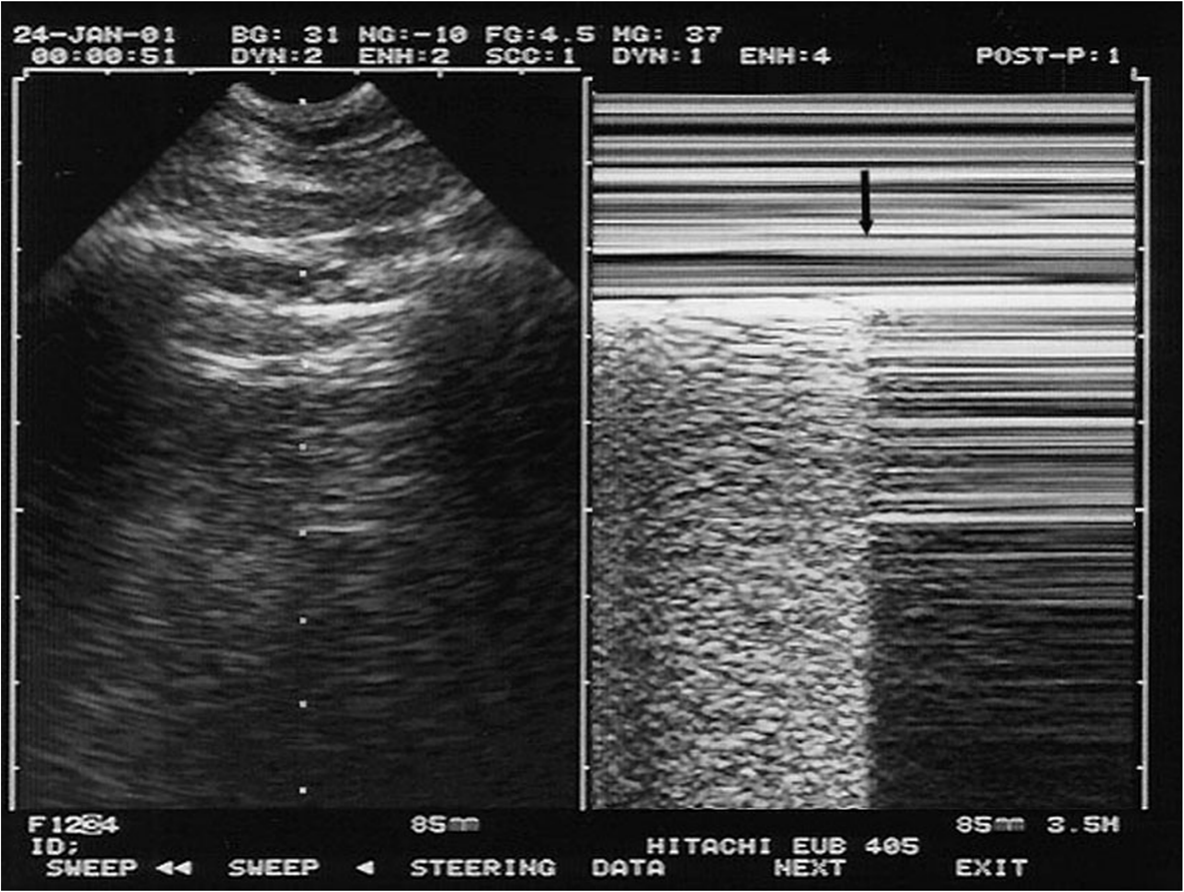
Pneumothorax and the lung point. A specific sign of pneumothorax. Real-time mode allows detection of the inspiratory increase in volume of the collapsed lung. When reaching the chest wall where the probe is laid, it makes a sudden change in the ultrasound image, from an A’-profile to an A- or B-profile usually. The change is sudden because (using an appropriate equipment, without average filters or time lag mainly) ultrasound is a highly sensitive method, able to detect subtle changes, such as the difference between free gas and alveolar gas. The left image shows the pleural line just before the visceral pleura appears. The right image shows (arrow) the very moment the visceral pleura has touched the parietal pleural. This sign has been called lung point (it can be seen along a line, but one point is sufficient for the diagnosis). Video visible at CEURF.net. Extract from “Whole body ultrasonography in the critically ill” (2010 Ed, Chapter 18), with kind permission of Springer Science.
